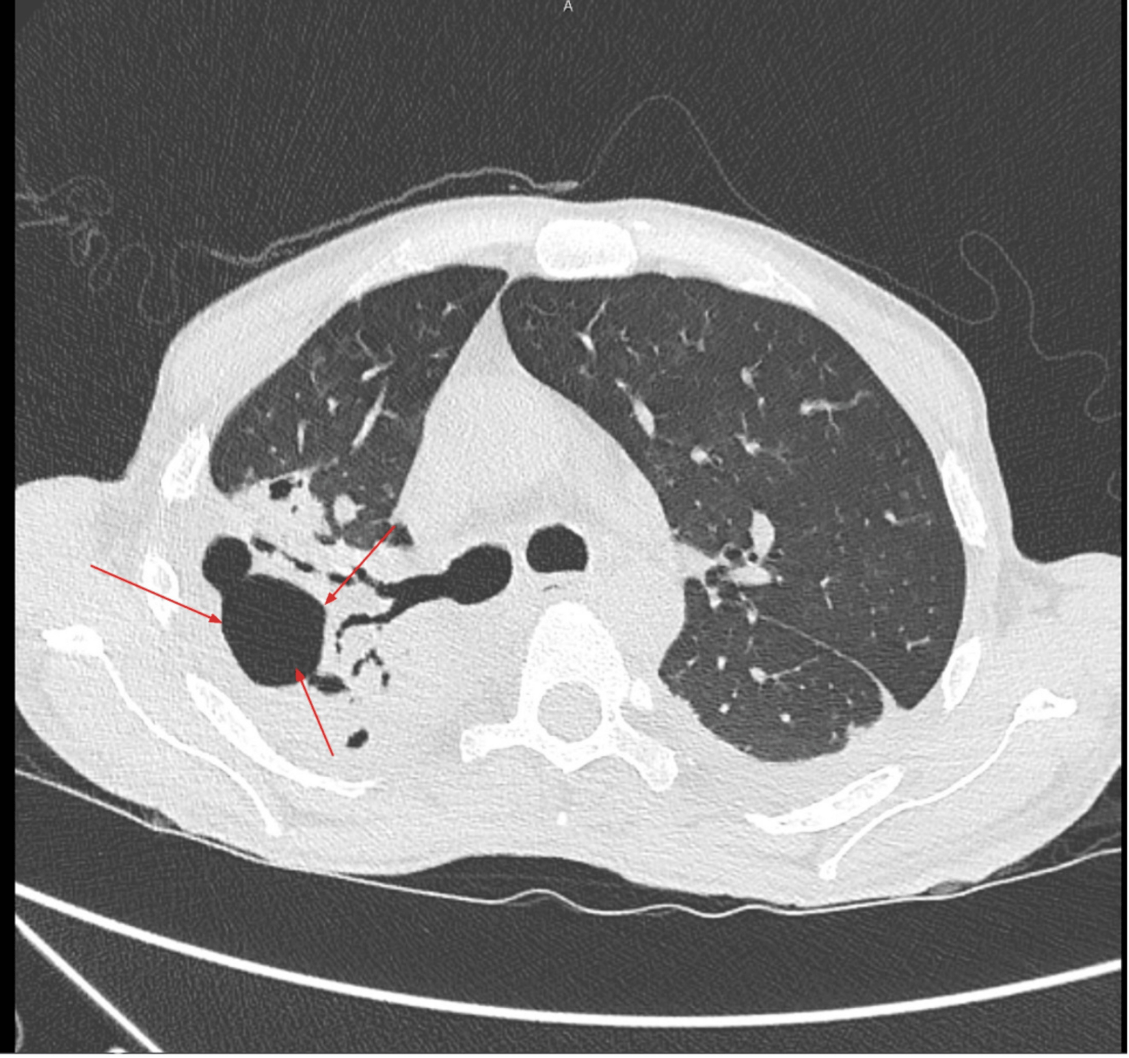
Axial chest CT shows a large, thick-walled cavitary lesion in the right upper lobe with multiple internal air-fluid levels and surrounding parenchymal consolidation
